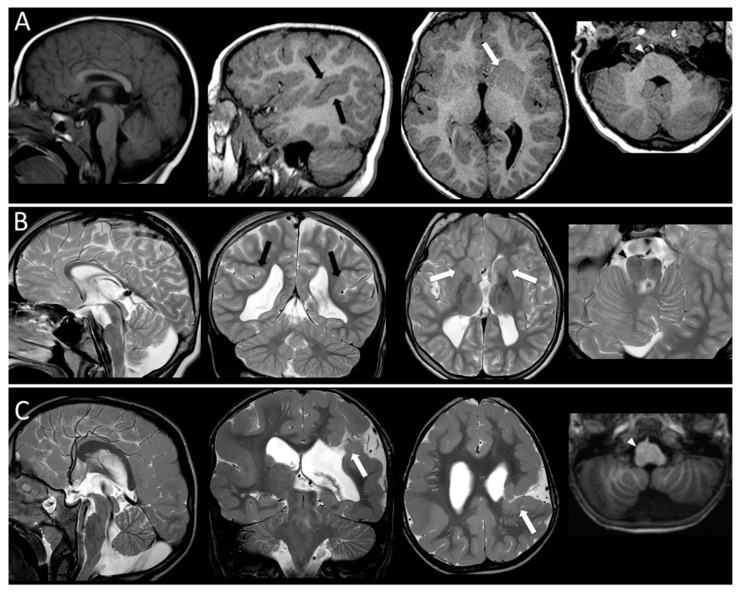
MR imaging findings in three patients. Patient A [P109418] has a mutation in *TUBB3* gene. T1-weighted images show a thin and short corpus callosum, perisylvian polymicrogyria (black arrow), a severe thinning/agenesis of the ALIC (white arrow) and brainstem asymmetry (arrowhead pointing at enlarged pons). Patient B [P45617] carries a mutation in *TUBA1A* gene. Thin corpus callosum, dysgyric cortex in perisylvian areas (black arrows), bilaterally thinned ALIC (white arrows) and pons asymmetry (arrowhead pointing at enlarged pons) are shown on T2-weighted images Patient C [P76712] carries a mutation in *TUBB2B* gene. He has a malformed corpus callosum and brainstem, with a thickened ponto-medullary junction and brainstem asymmetry (arrowhead pointing at enlarged medulla). He has also a complex cortical malformation with diffuse bilateral polymicrogyria and schizencephaly in the left hemisphere (arrows).
